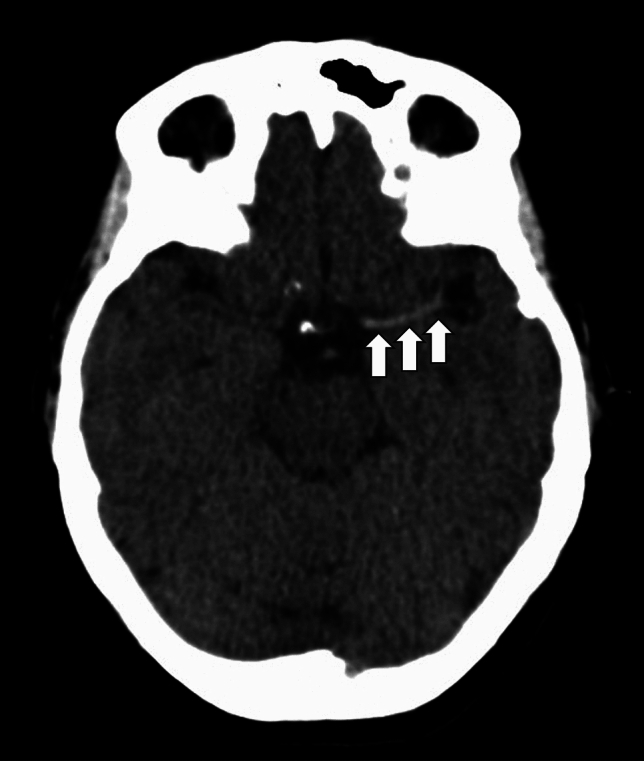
A non-contrast CT head demonstrating a hyperdense left middle cerebral artery indicative of thrombus (white arrows)
